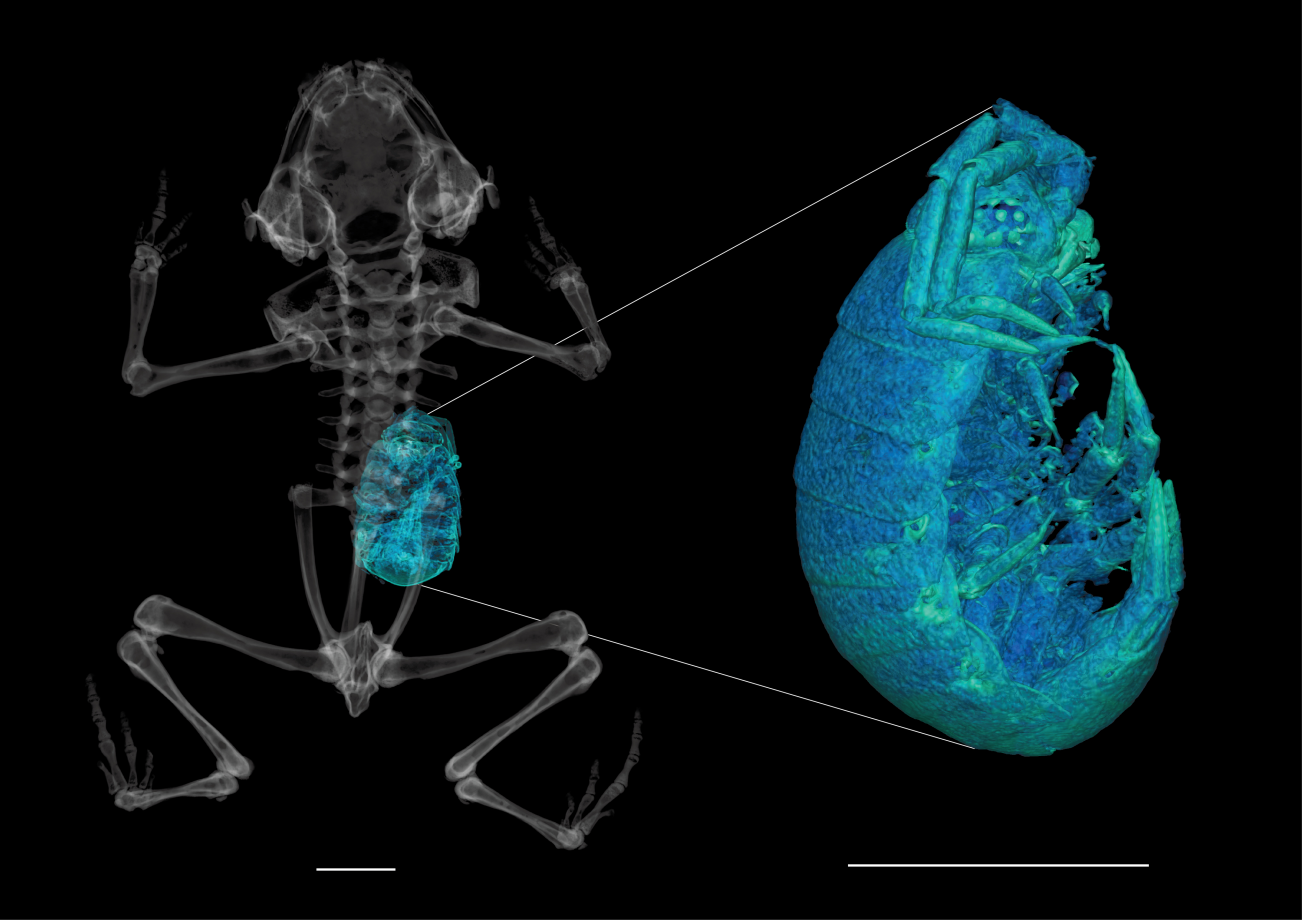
High-resolution CT scan of paratype of *Brachycephalus curupira* (MHNCI 10285) revealing a single isopod (enlarged at right) in the stomach. Scale bars equal 2 mm.
